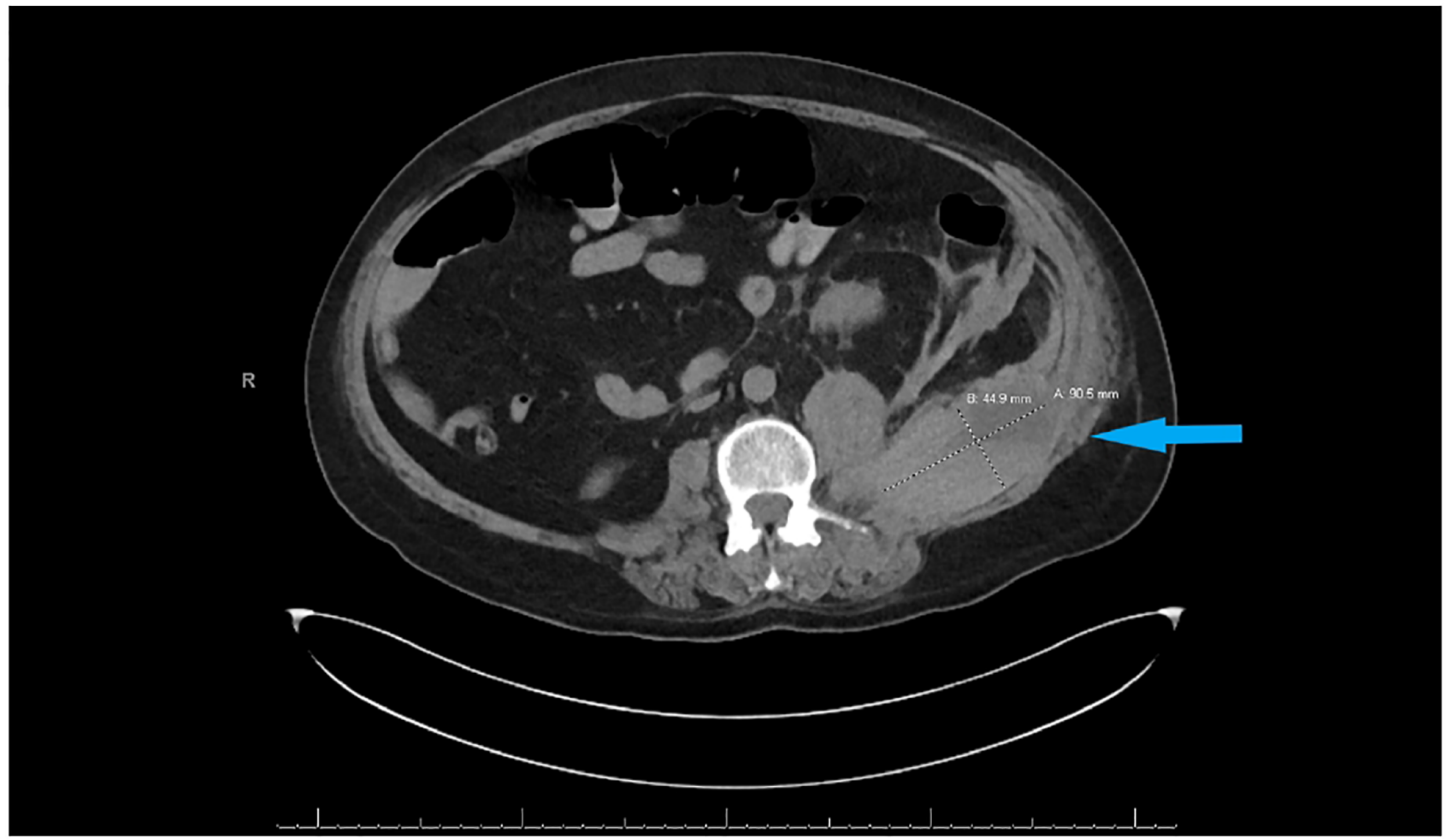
CT abdomen and pelvis without contrast showing left retroperitoneal hemorrhage behind the left psoas muscle (blue arrow). Subcutaneous heparin was discontinued, and the patient received 2 units of packed red blood cell transfusion (PRBC) and underwent angiogram with gel foam embolization of the left L3 lumbar artery. Since the patient continued to have a persistent drop in hemoglobin to 7.9 g/dl 2 days after embolization, he was taken back to the operating room for a second embolization. Between the first and second embolization, he received desmopressin 4 mcg IV once as his BUN was 118 mg/dl. Although he underwent a second embolization, point-of-care thromboelastography (TEG) with platelet mapping in the presence of heparinase was performed as the suspicion of uremic platelet dysfunction was high. It showed decreased platelet aggregation (11.9) and increased inhibition (68.1), as shown in Figure 3A confirming our diagnosis. Hemodialysis was initiated, and after three dialysis treatments, TEG was repeated, showing an improvement in the parameters (Figure 3B).
